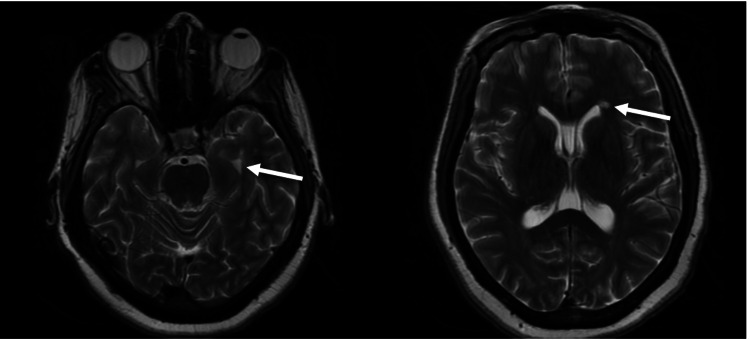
T2-weighted magnetic resonance images of the head. Horizontal cuts show numerous periventricular white matter hyperintensities with involvement of the temporal horns, especially the left, giving evidence of demyelinating lesions.
